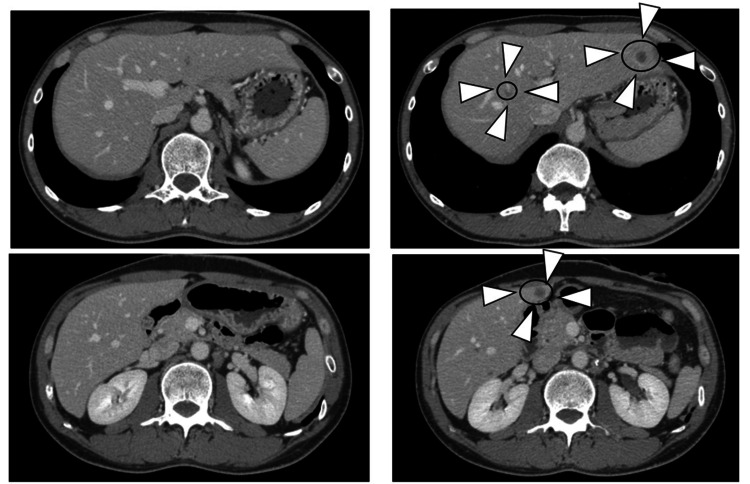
Abdominal CT findings of the patient. Comparison between four months before (left) and at the starting of the triplet regimen (right). Multiple liver metastases appeared and some lymph node metastases increased in size.
